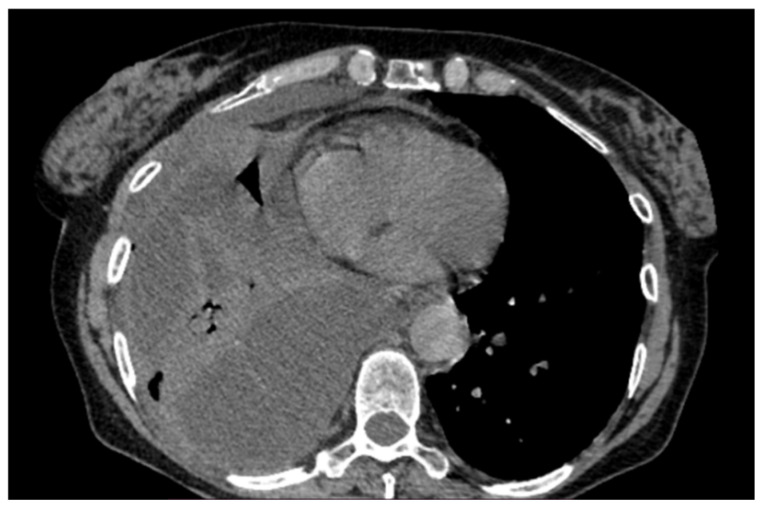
Large right pleural effusion on chest CT scan.
